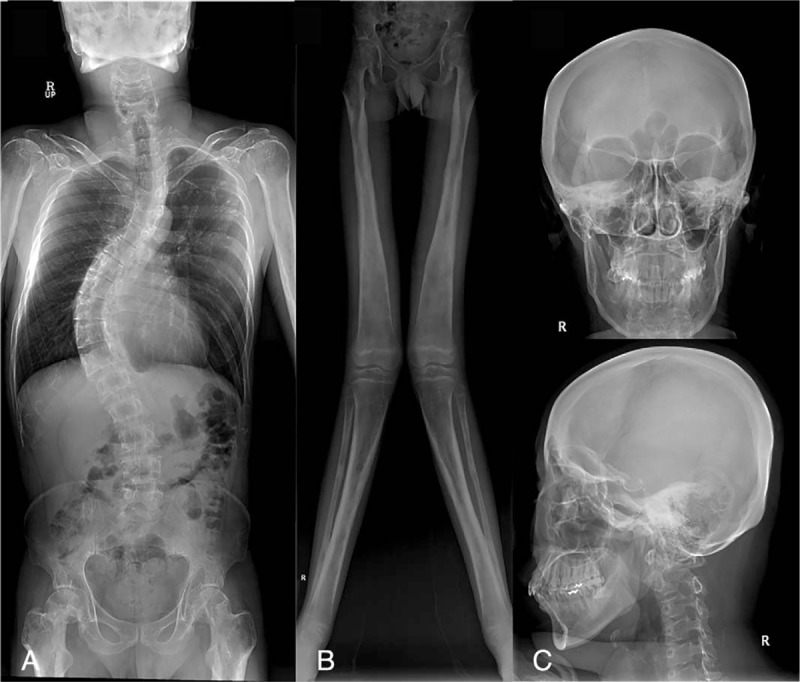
(A) Spine radiograph of patient 2. Note the severe thoracolumbar spine scoliosis at the T3–L3 level. (B) Lower-extremity radiographs of patient 1. Bilateral symmetrical hyperostosis and endostosis of the diaphysis were noted in both femurs, tibias, and fibulas. (C) Skull radiographs of patient 3 showing bilateral sclerotic bony enlargement in the skull base and calvarium.
